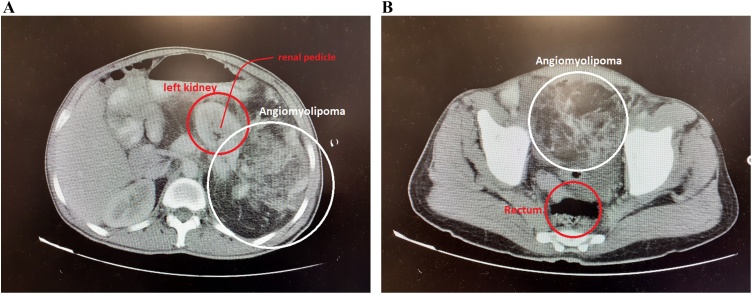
Computed Tomography Scan at the time of diagnosis. A- representing the origin of larger tumor from left renal pedicle B- representing the huge mass extended to pelvis
